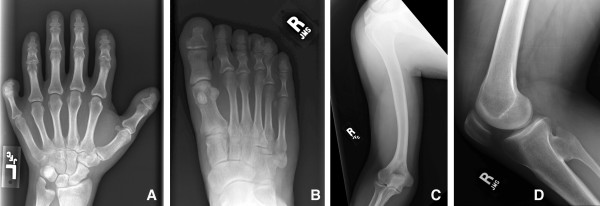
Radiographs of patient IV-9. A) Coned epiphysis of the left second middle phalanx and prominent styloid process of the ulna B) Irregular notched tip of the distal phalanx of the hallux C) Bowing of the right humerus D) Fusion of the right proximal tibia and fibula.
